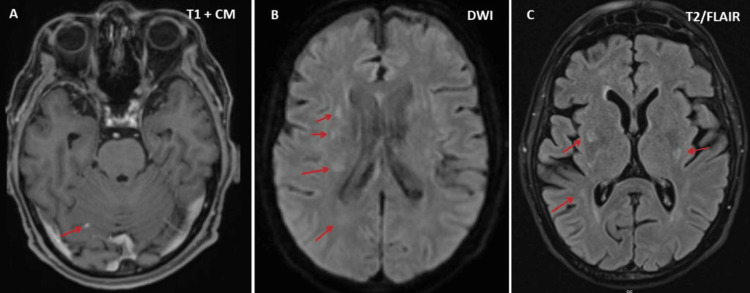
Cerebral MRI showing multiple parenchymal lesions, appearing faintly hyperintense on T2-weighted images (panel C), exhibiting mild diffusion restriction (panel B), and faint contrast enhancement (panel A). The largest supratentorial lesion is located in the periventricular white matter of the right frontal lobe (arrow), with a further lesion in the white matter of the left frontal lobe. Smaller lesions are present subcortically in the left temporal lobe and in the right fronto-basal lobe (arrows)
